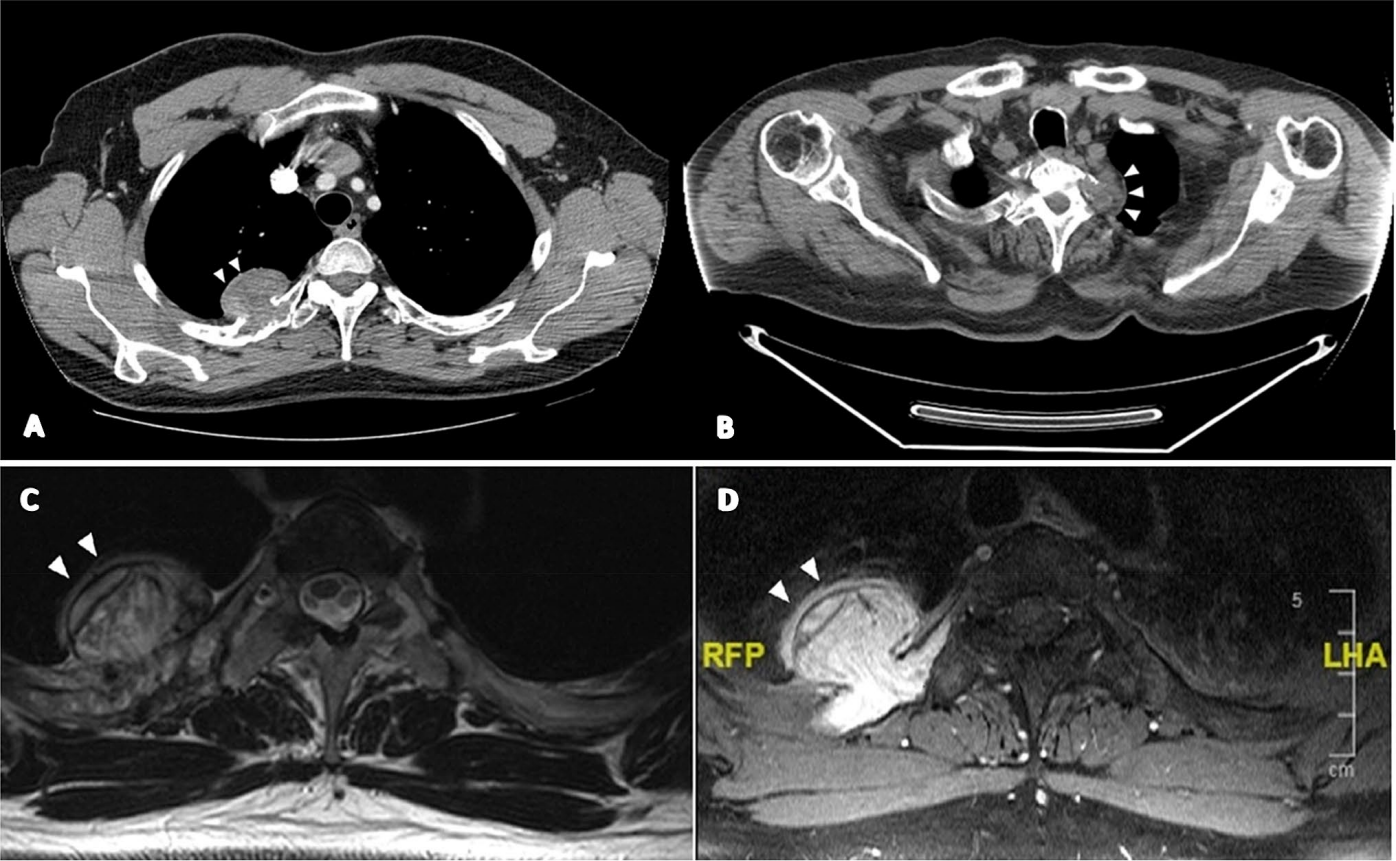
47-year-old man with a PMT of the 4th rib. (A, B) Axial CT demonstrates a well-defined rounded dense lesion with a mildly calcified internal matrix. (C) Axial T2 weighted spin-echo MR image shows a lesion of the 4th rib with mixed hyper- and hypointense signal, well-defined borders, cortical destruction, and extension into the extrapleural fat. (D) Axial T1 weighted fat suppressed contrast enhanced image shows intense uniform enhancement of the lesion. The non-enhancing margins (arrowheads) are areas of calcification
